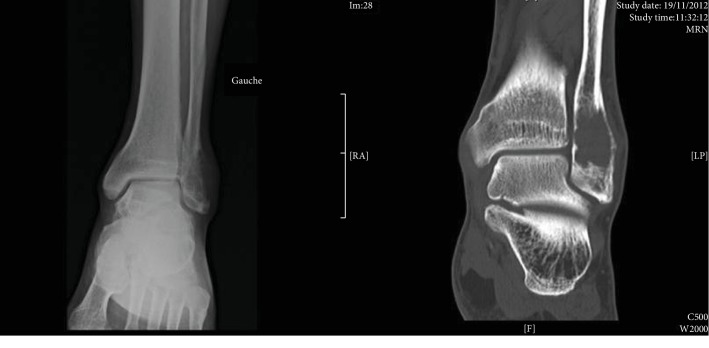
Preoperative radiological and CT-scan evaluations.
